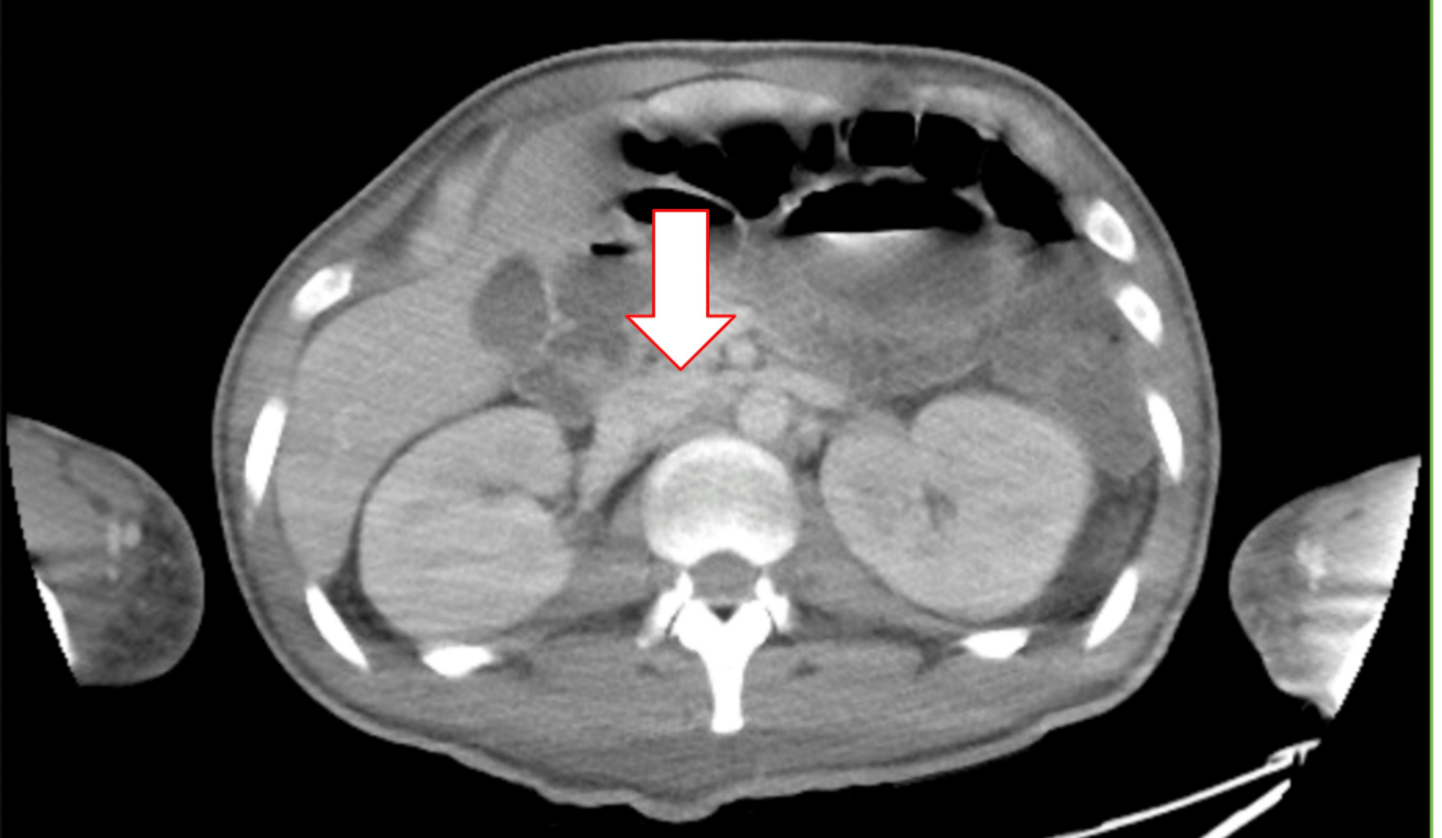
CT abdomen and pelvis (axial view), obtained prior to IAA results returning positive, with the arrow pointing to the pancreas; no insulinoma is present. IAA: Insulin autoantibodies.
